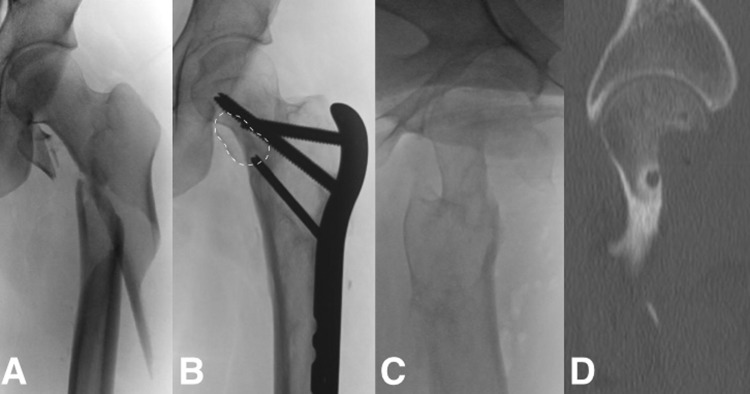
Initial presentation, subtrochanteric fracture with lesser trochanter avulsion (A). Adequate consolidation of the subtrochanteric fracture with evident medial displacement of the lesser trochanter (dotted line) (B), evident femoral neck junction resection after plate removal (C), resection close to 50% confirmed by CT (D).
